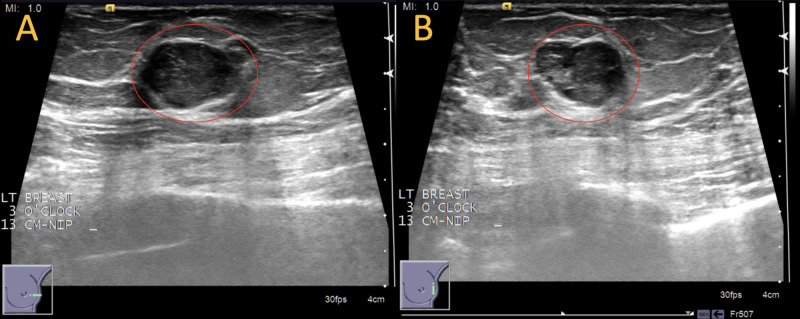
Left breast diagnostic ultrasound Images show left breast oval mass with circumscribed margins measuring 15 mm (red circles). An ultrasound was performed in January 2019.
